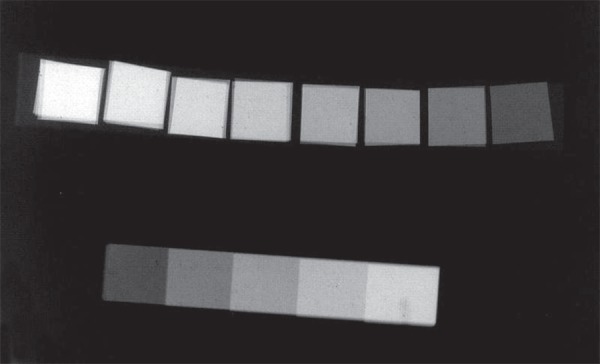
Radiograph of the prototype Cu stepwedge formed according to the x-ray attenuation
